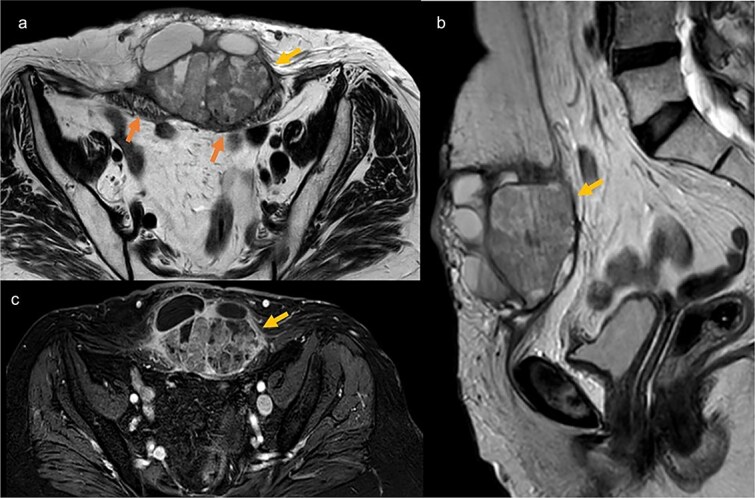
Pelvic MRI in axial T2 (a), sagittal T2 (b), and axial T1 post-contrast (c), showing a well-defined mass at the trocar site, infiltrating the rectus abdominis muscles. The mass has regular contours, heterogeneous content with both fluid and tissue components, and exhibits enhancement after contrast injection.
